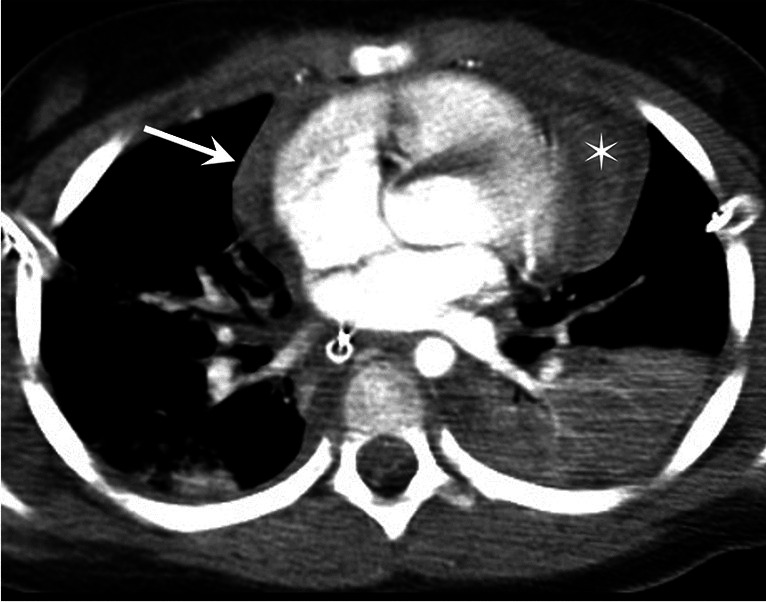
A 12-year-old boy with hemopericardium. Axial contrast-enhanced computed tomography of the thorax demonstrates high density pericardial fluid (*asterisk*) consistent with hemopericardium. Note also a small mediastinal hematoma (*arrow*) and consolidation/atelectasis of the posterior lungs, more confluent on the left. Courtesy of Dr. David Manson from The Hospital for Sick Children, Toronto, Canada
